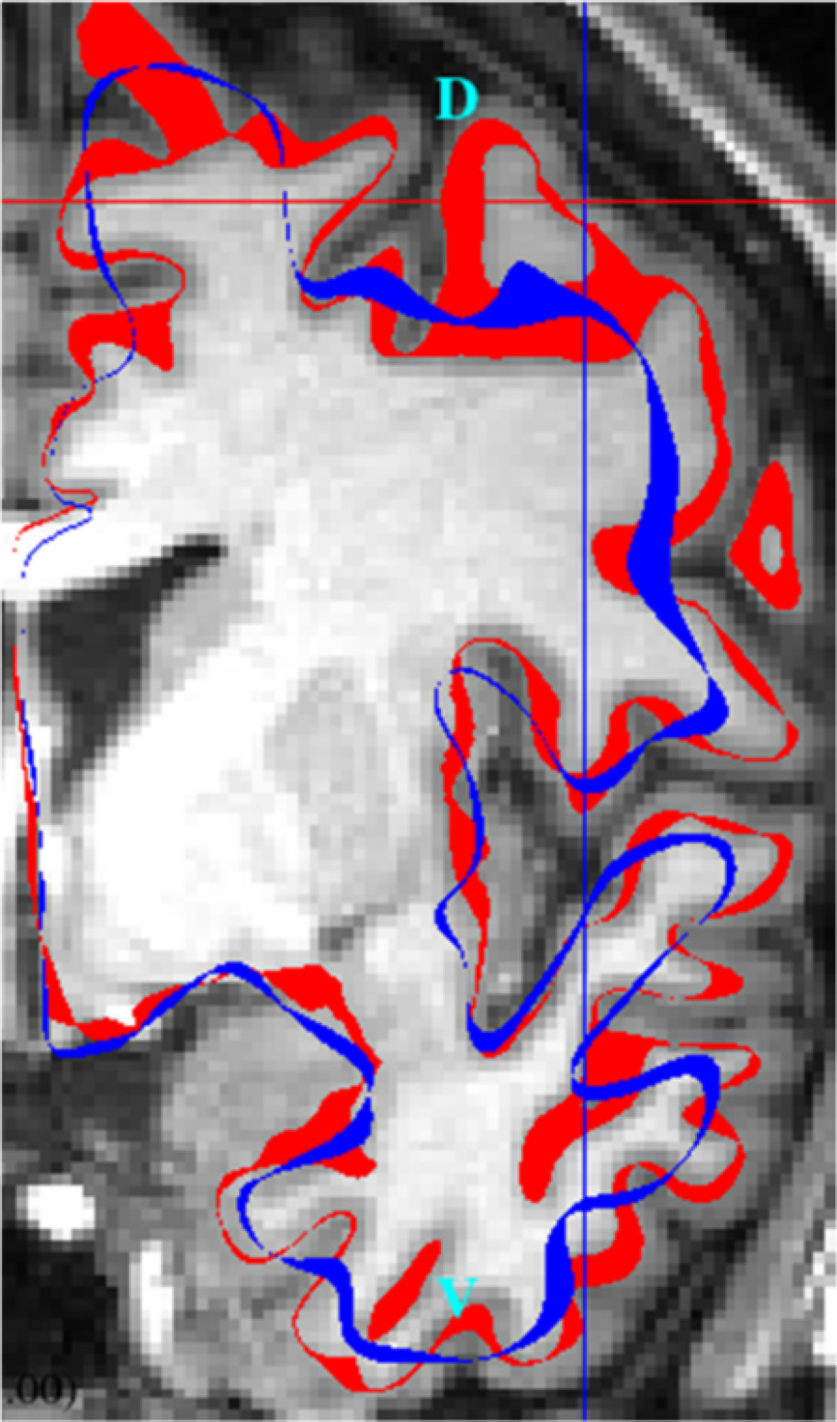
A Representation of Cortical Folding Measures. This is a coronal MRI slice of patient RD05. The red line represents the subject’s midthickness surface in Montreal Neurological Institute space. The blue line represents the HCP196 mean midthickness surface. D = dorsal; V = ventral
